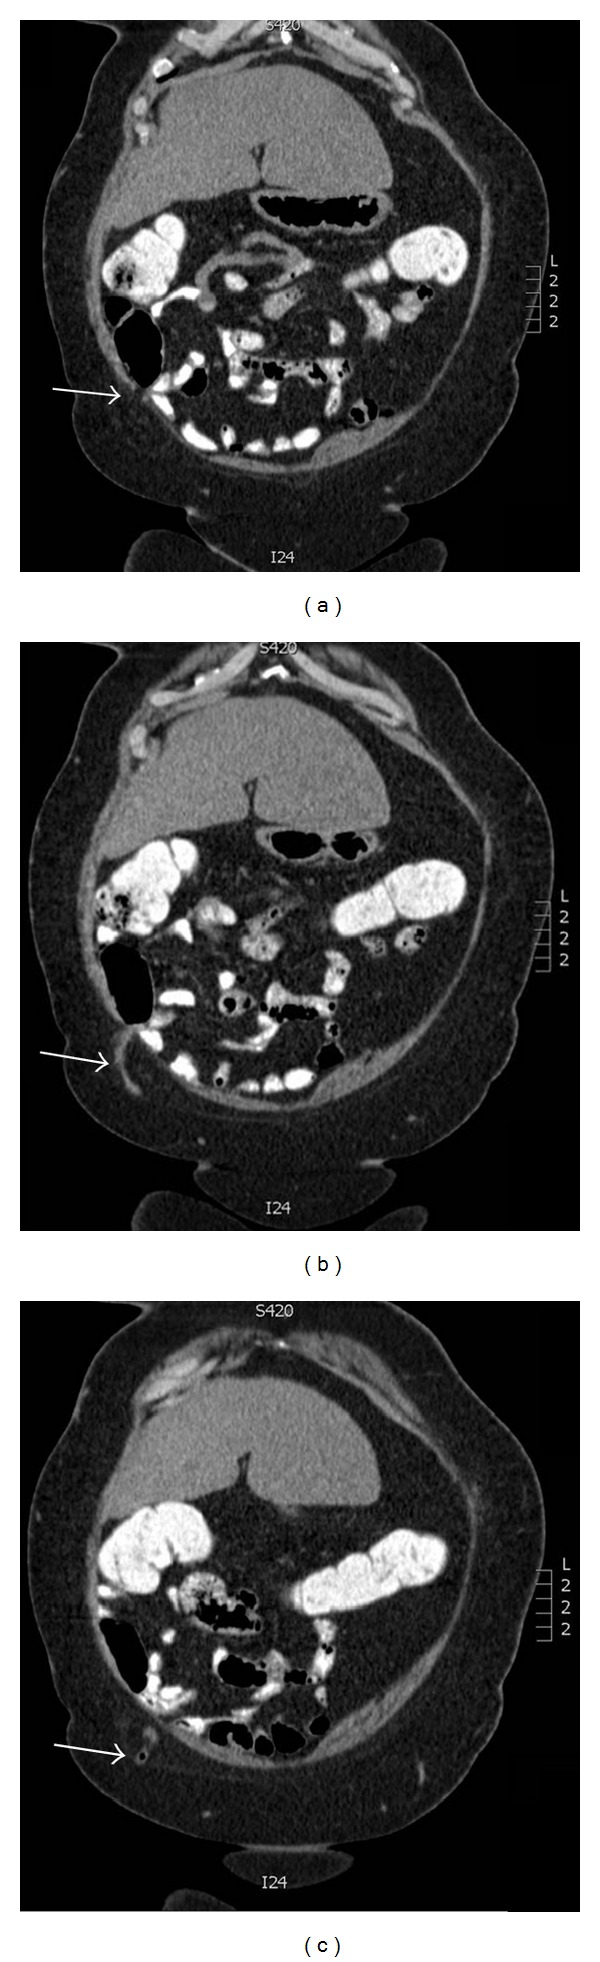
CT findings of the subcutaneous vermiform appendix. (a) Arrow indicating hernia orifice. (b) Arrow indicating subcutaneous position of vermiform appendix. (c) Arrow indicating a bubble of air in the tip of the vermiform appendix.
